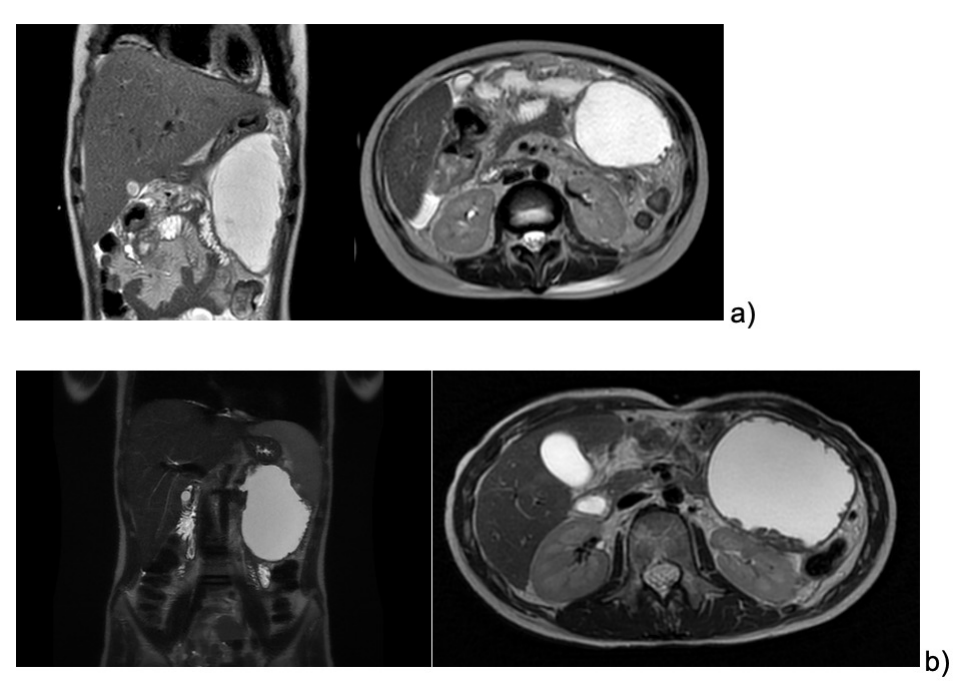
a) Abdominal MRI 14 days after APP; b) Cholangio MRI 3 months after APP
